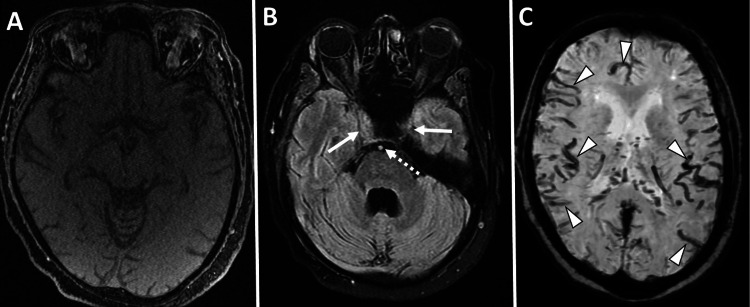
(A) Axial image of 3D time-of-flight magnetic resonance angiogram at the level of Sylvian fissures demonstrates a complete absence of flow-related signal within the intracranial circulation. (B) Axial fluid-attenuated inversion recovery MRI of the brain (inversion time 2500, repetition time msec/echo time msec, 9000/107, 5-mm section thickness) demonstrates loss of vascular signal void in the intracranial bilateral carotid arteries (solid white arrow) and basilar artery (dashed white arrow). (C) 3D susceptibility-weighted angiography (SWAN) imaging demonstrates serpiginous low signal intensities likely due to an intravascular buildup of deoxyhemoglobin and stagnant blood flow within most of the intracranial arterial and venous circulation (solid white arrowheads).
